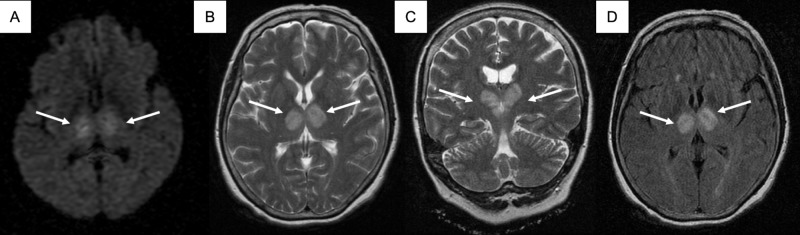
Case MRI images. (A) Axial MRI in DWI sequence showing bilateral thalamic restricted diffusion. (B-C) Axial and coronal section T2 sequence with bilateral thalamic hyperintensities with extension to the midbrain. (D) Axial FLAIR sequence in which bilateral thalamic hyperintensities are seen. DWI: Diffusion-weighted imaging; FLAIR: Fluid-attenuated inversion recovery; MRI: Magnetic resonance imaging.
